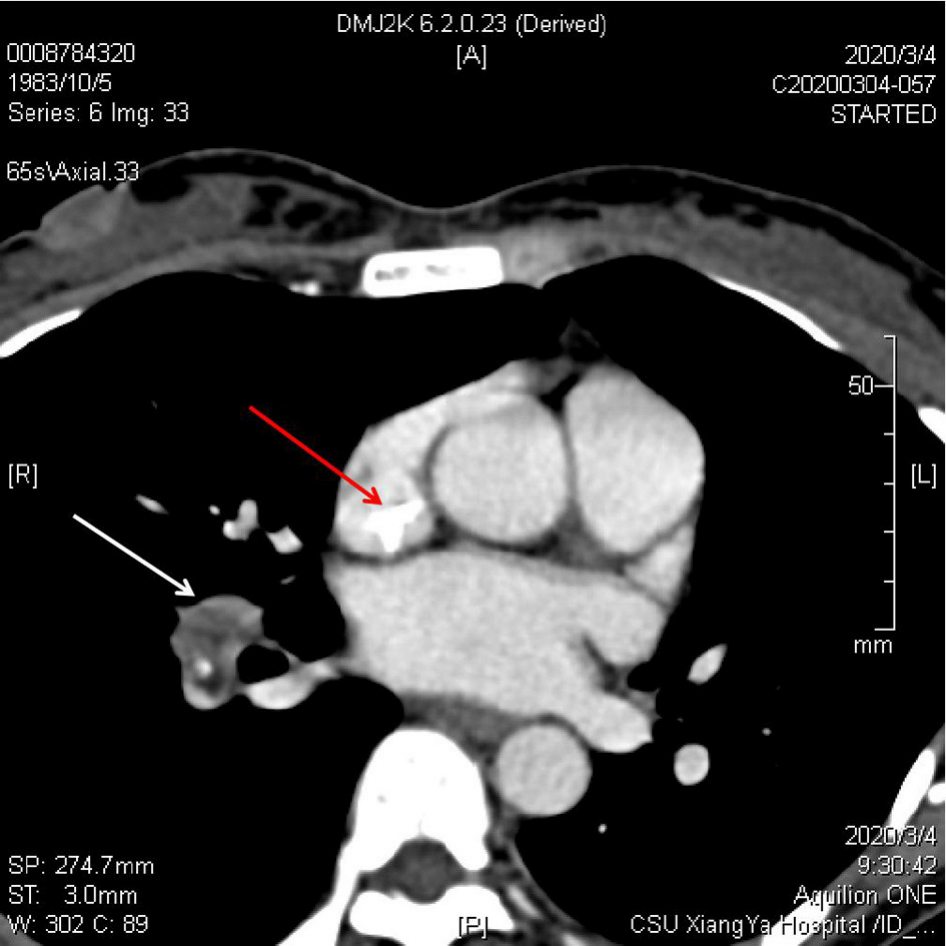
The CT findings of the patient showed calcified thrombus in superior vena cava and pulmonary artery thrombosis.
